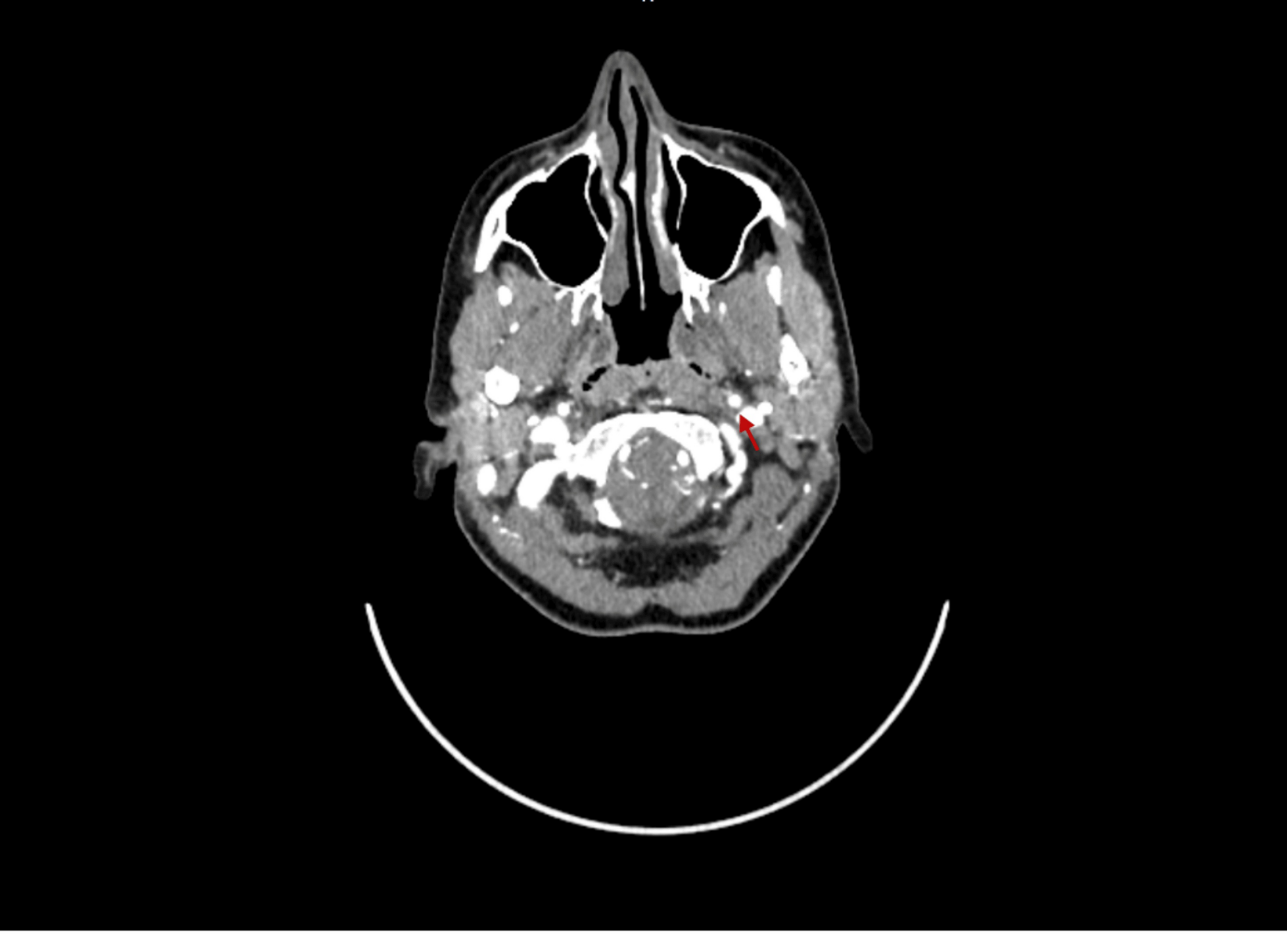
Follow-up CT angiography after four months Follow-up CT angiography after four months demonstrated the resolution of the previously noted circumferential narrowing and mural thickening of the left internal carotid artery (ICA), consistent with resolved intramural haematoma. The lumen is now of normal calibre with no residual thrombus or contrast flow limitation.
